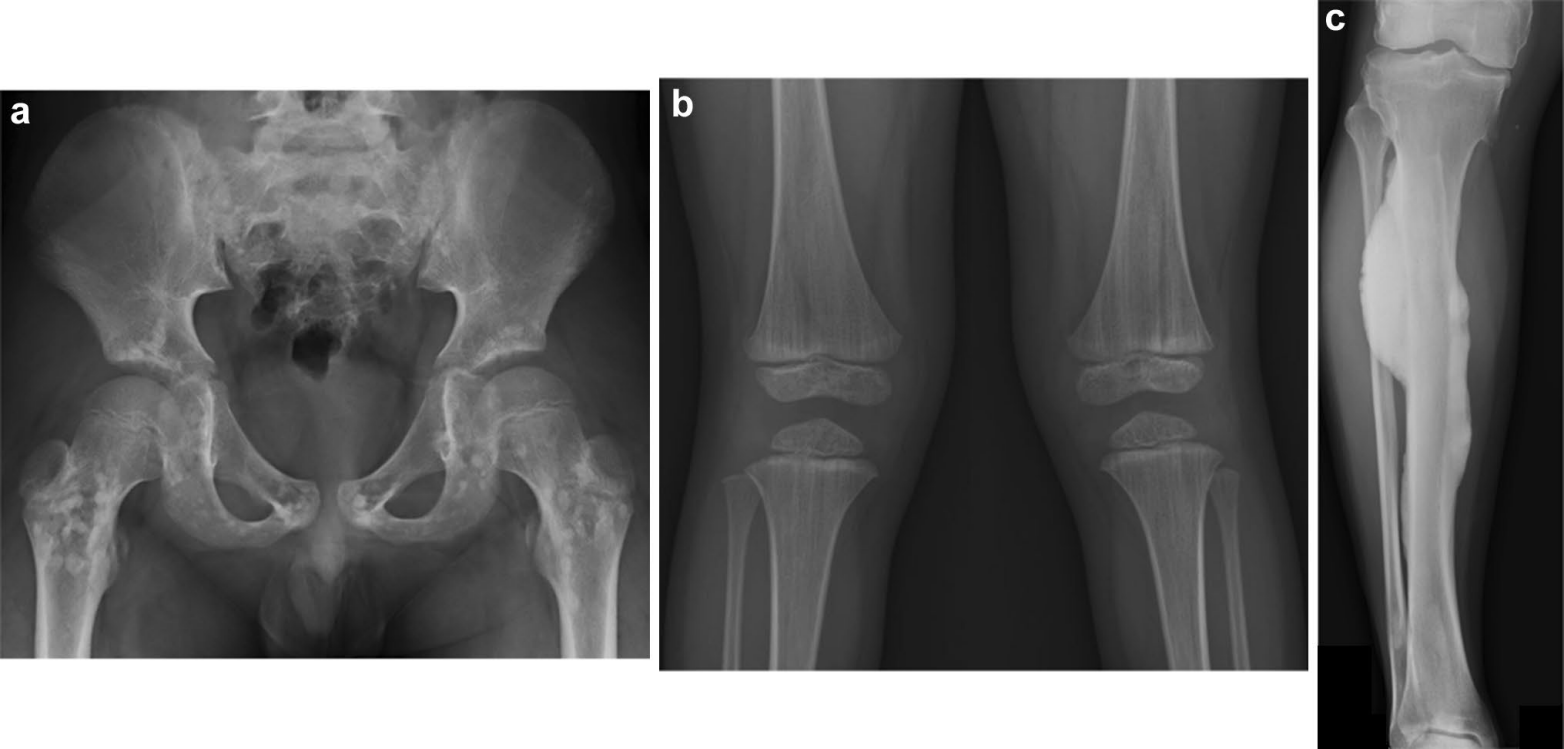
a A 10-year-old boy with osteopoikilosis. Radiograph shows small foci of bone sclerosis in different sizes and shapes (round, oval, lenticular) located in the pelvis and bilateral proximal femora. b A 4-year-old girl with osteopathia striata. Radiograph shows longitudinal striations in the distal femoral and proximal tibial metaphyses. c A 75-year-old male with melorheostosis. Radiograph shows dripping candle wax-like, eccentric hyperostoses distributed along the tibia
